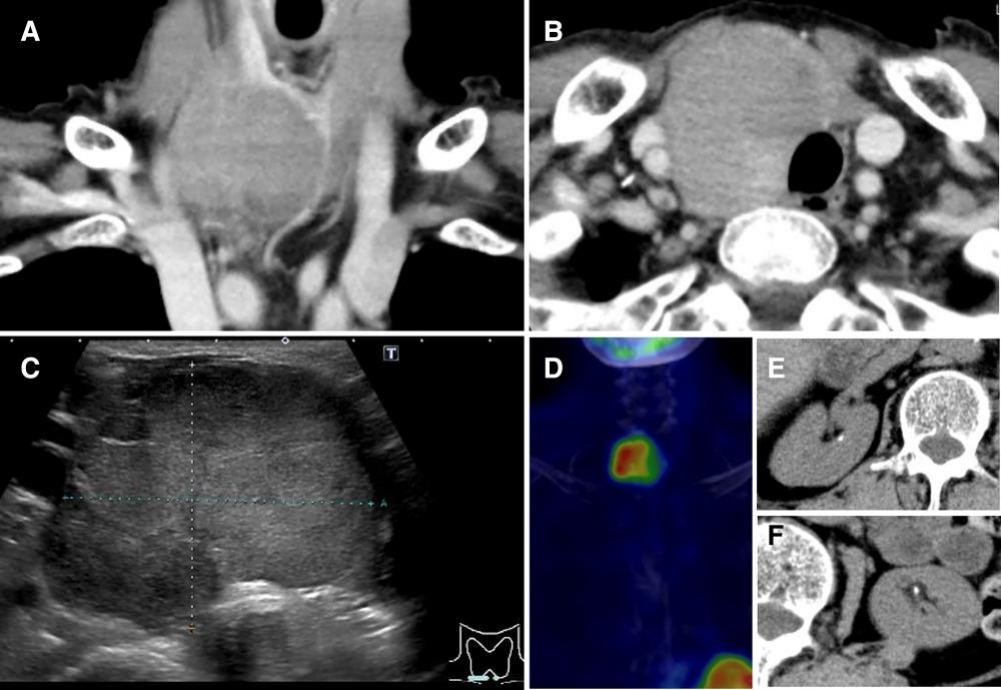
Twenty months after presentation, the tumor became almost totally solid on computed tomography (CT) (A, B) and ultrasonography (C) and was positive for Tc-99 m sestamibi scintigraphy (D). Small renal calculi were noted on CT (E, F).
